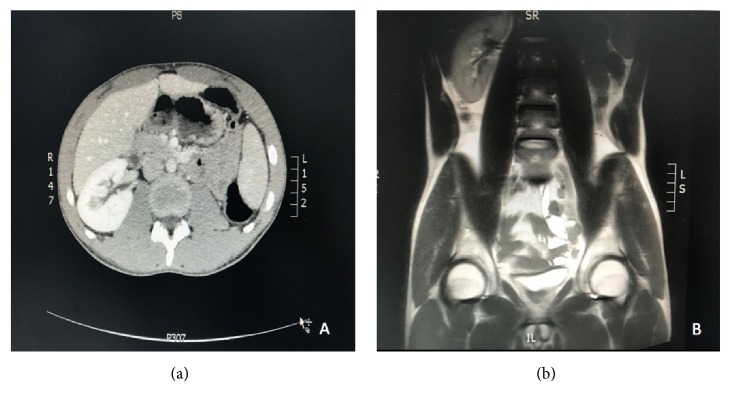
(a) CT axial section showed left renal agenesis. (b) MRI coronal section showed left renal agenesis.
